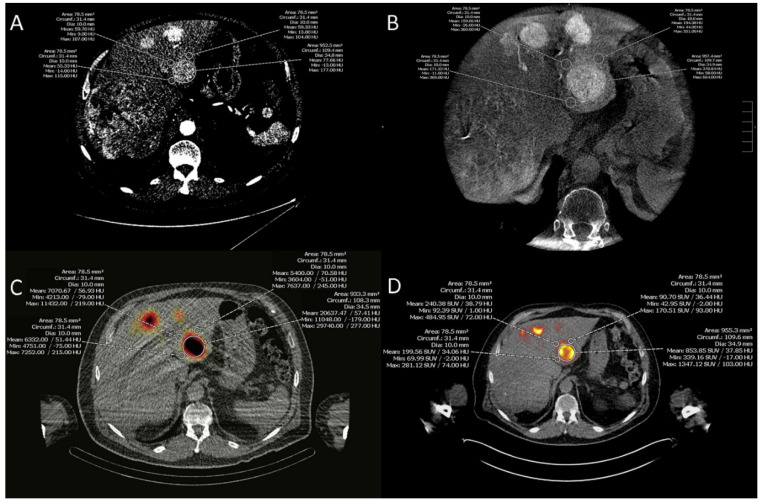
Image analysis. A region of interest was drawn on the tumor and three regions of interest were placed on the adjacent liver tissue in equal positions on arterial phase multi-detector tomography (A), parenchymal phase C-arm computed tomography (B), Technetium^99^m-macroaggregates of human serum albumin single-photon emission computed tomography and computed tomography (C), Yttrium^90^ positron emission tomography and computed tomography (D), mean hounsfield units, counts, and becquerel/milliliter were recorded.
